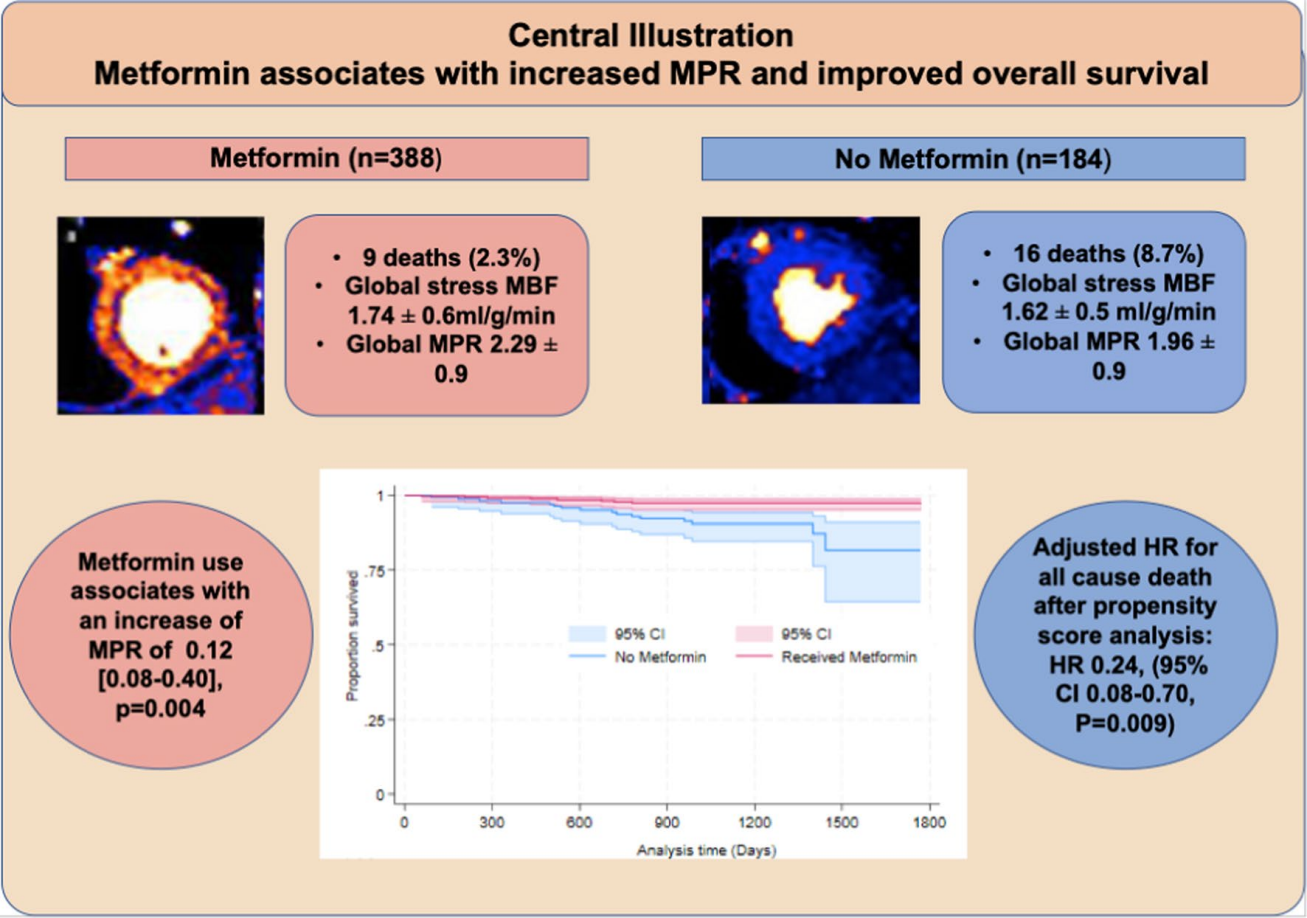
This retrospective, multicentre, longitudinal cohort study showed that in patients with T2DM, metformin use is associated with higher MPR on quantitative perfusion cardiovascular magnetic resonance imaging and improved all cause survival.
